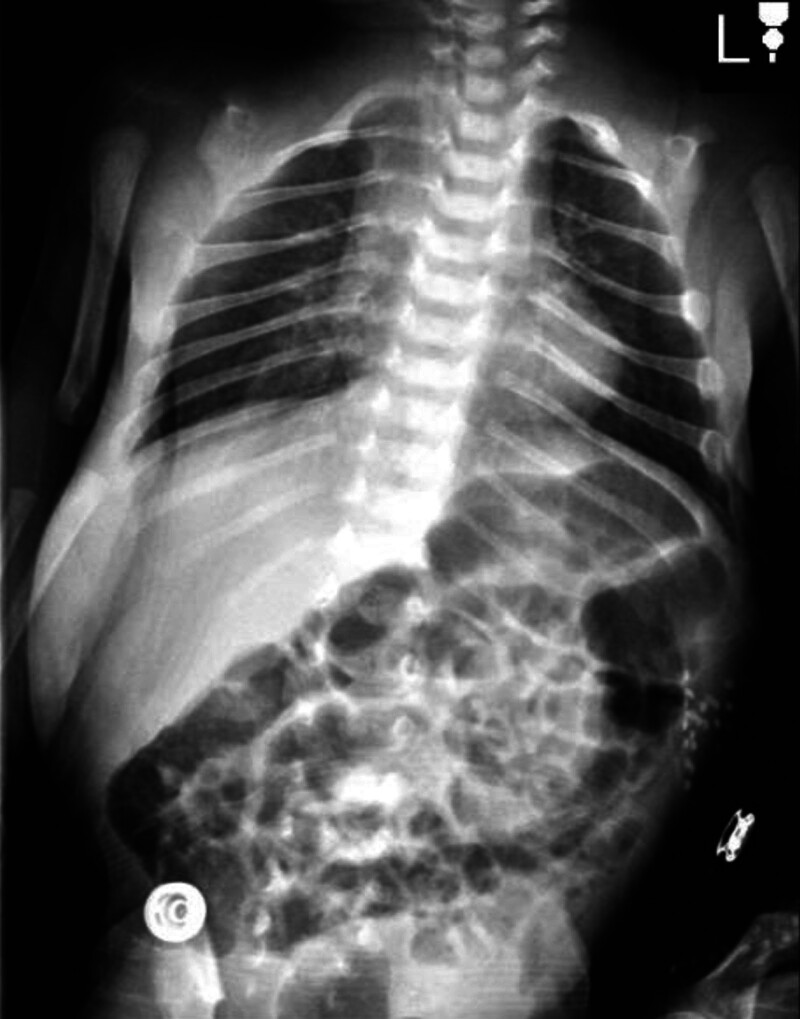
Chest and abdomen X-ray showed the absence of both clavicles.
